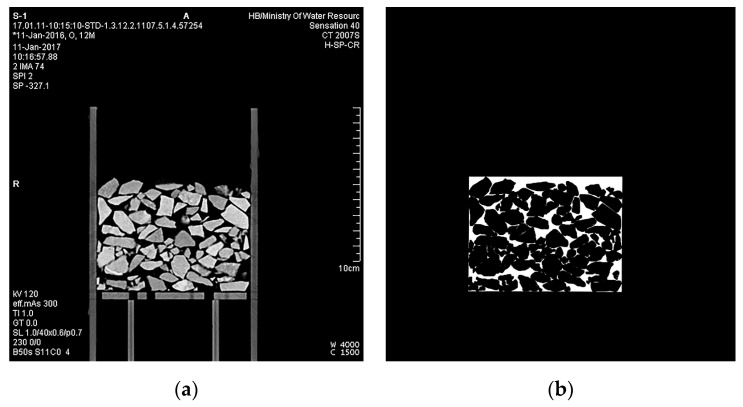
Image segmentation based on the SVM. (a) The original CT scanning image. (b) The image after segmentation.
